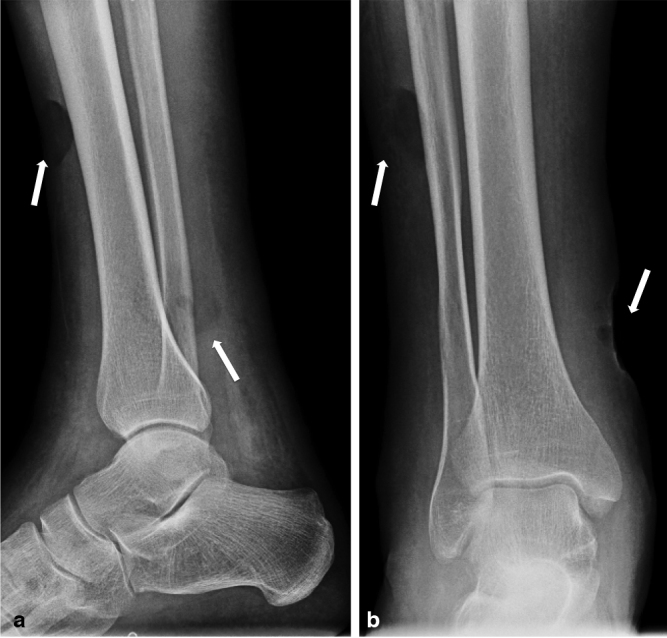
(a) Lateral and (b) frontal radiographs of the right leg demonstrating diffuse thickening and reticular, micronodular infiltration of the subcutaneous soft tissues and the presence of two cutaneous ulcerations (arrows) without evidence of soft-tissue calcification or osteomyelitis.
